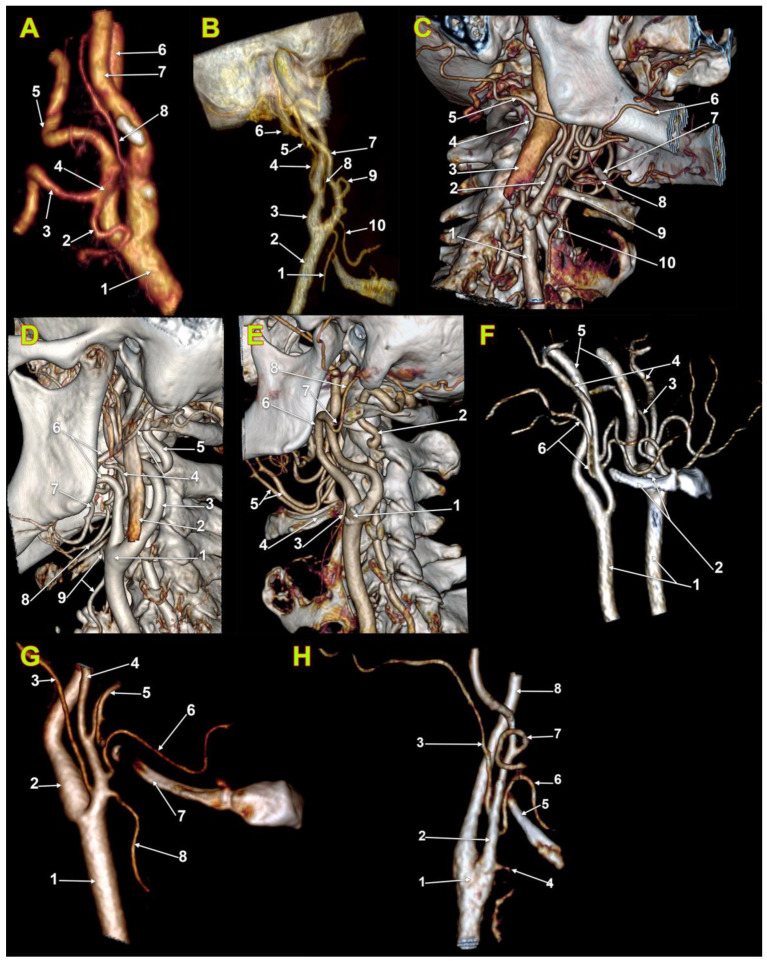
Computed tomography angiograms, three-dimensional volume renderings. (A). Origin of the occipital artery from the internal carotid artery. Right side. Postero-medial view. 1. common carotid artery; 2. lingual artery; 3. facial artery; 4. linguofacial trunk; 5. external carotid artery; 6. internal jugular vein; 7. internal carotid artery; 8. occipital artery. (B). Origin of occipital and ascending pharyngeal arteries from internal carotid artery. Right side. Infero-lateral view. 1. superior thyroid artery; 2. common carotid artery; 3. internal carotid artery; 4. occipital artery; 5. posterior auricular artery; 6. internal jugular vein; 7. internal carotid artery; 8. ascending pharyngeal artery; 9. facial artery; 10. lingual artery. (C). Infragonial (type 3) origin of the right occipital artery. Right side. Infero-lateral view. 1. common carotid artery; 2. external carotid artery; 3. internal jugular vein; 4. vertebral artery; 5. occipital artery; 6. facial artery; 7. contralateral ceratohyal bone; 8. lingual artery; 9. greater horn of hyoid bone; 10. superior thyroid artery. (D). Gonial (type 4) origin of the left occipital artery. Left side. Postero-infero-lateral view. 1. carotid bifurcation; 2. internal jugular vein; 3. internal carotid artery; 4. occipital artery; 5. vertebral artery; 6. external carotid artery; 7. facial artery; 8. lingual artery; 9. superior thyroid artery. (E). Origin at supragonial level (type 5) of the occipital artery. Left side. Infero-lateral view. 1. carotid bifurcation; 2. vertebral artery; 3. superior thyroid artery; 4. left greater horn of hyoid bone; 5. lingual arteries; 6. external carotid artery; 7. posterior auricular artery; 8. occipital artery. (F). Origin at the hyoid level (type 2) of the right occipital artery. Bilateral asymmetry of occipital artery origin. Right side. Antero-lateral view. 1. common carotid arteries; 2. greater horns of hyoid bone; 3. left occipital artery (suprahyoid origin); 4. external carotid arteries; 5. internal carotid arteries; 6. right occipital artery (hyoid origin). (G). Infrahyoid (type 1) origin of the occipital artery, at the same level with the origin of the superior thyroid artery. Right side. Antero-lateral view. 1. common carotid artery; 2. internal carotid artery; 3. occipital artery; 4. external carotid artery; 5. facial artery; 6. lingual artery; 7. greater hyoid horn; 8. superior thyroid artery. (H). Infrahyoid (type 1) origin of the occipital artery, above the level of the lingual artery origin. Right side. Antero-infero-lateral view. 1. carotid bifurcation; 2. external carotid artery; 3. occipital artery; 4. superior thyroid artery; 5. greater horn of the hyoid bone; 6. lingual artery; 7. facial artery; 8. internal carotid artery.
